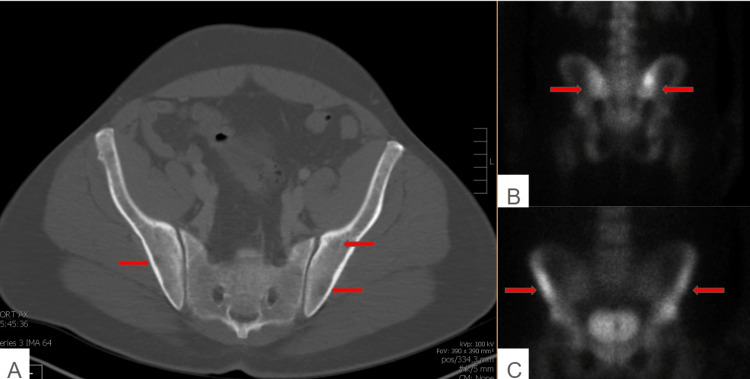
CT of the abdomen/pelvis showed a mottled appearance of the iliac crest (A). A nuclear medicine bone scan showed atypical uptake in bilateral periacetabular region (B,C) CT=Computed tomography, NM = Nuclear Medicine
